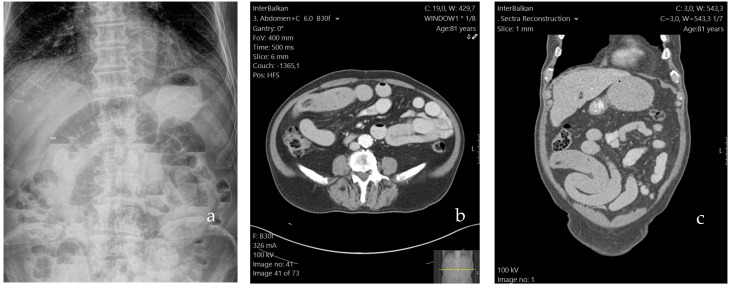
The patient’s preoperative abdominal X-ray (a) and CT scan; axial (b) and coronal (c) plane.
